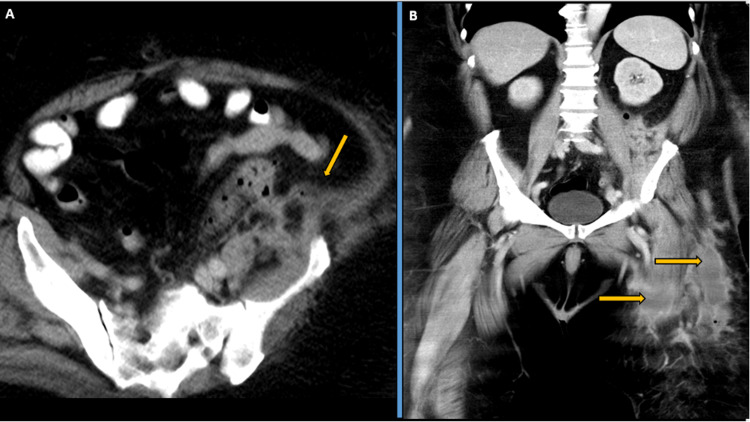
Day 18 CT (A) Axial contrast-enhanced computerised tomography (CECT) pelvis image: yellow arrows showing persistence of the fistulous tract from the left-sided descending colon extending to the left-sided retroperitoneum overlying the left iliopsoas. (B) Coronal CECT image with yellow arrows showing interval re-formation of the left thigh abscess with a liquified component.
